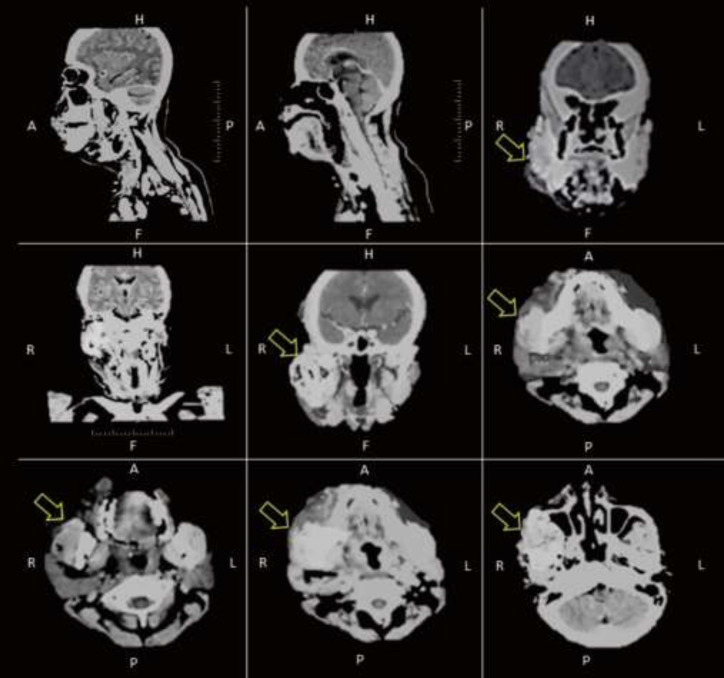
CT-scan imaging showed submandibular abscess with expansion to right parotid space, bilateral parapharyngeal space, epiglottis, retropharyngeal space, and upper-right neck area with prominent lymph nodes (yellow arrow)
